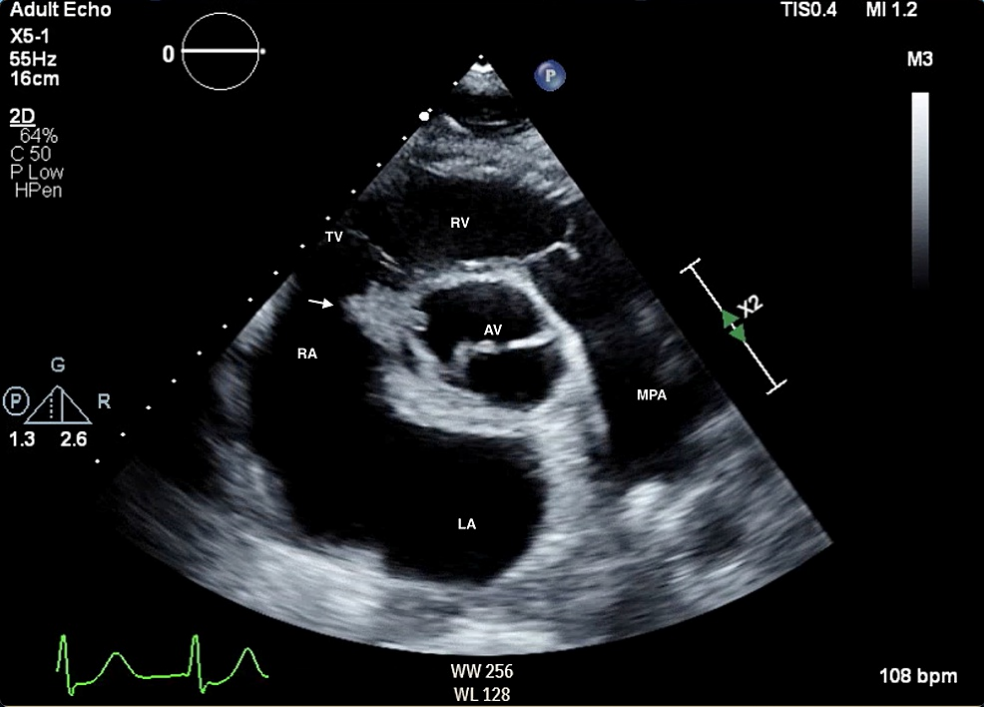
Transthoracic echocardiogram, parasternal short-axis view Demonstrates bicuspid aortic valve with a complex vegetation involving the aortic root and extending into the right atrium abutting the tricuspid valve (arrow). RV: right ventricle, TV: tricuspid valve, RA: right atrium, AV: aortic valve, LA: left atrium, MPA: main pulmonary artery.
